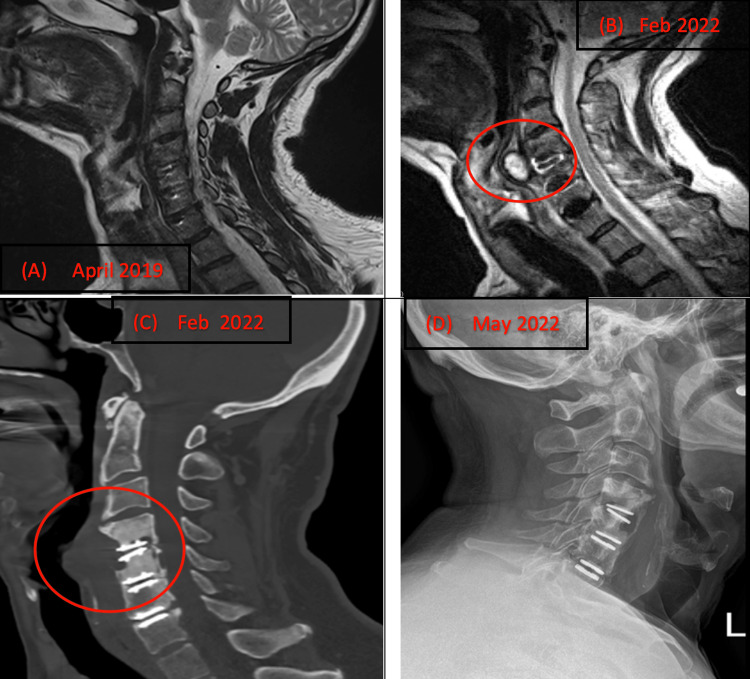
Images showing progressive changes seen within three years. (A) MRI of C4-C5 level shows sclerosis; (B) Cyst (red circle) is seen centrally anterior to the C4-C5 measuring 2.1 cm x 2.7 cm x 1.7 cm, right paracentral to the C4-C5 measuring 2.1 cm x 1.2 cm x 1.2 cm and left paracentral to the C3-C4 measuring 2.3 cm x 1.2 cm x 1.1 cm (not shown in the figure). (C) Mass effect on hypopharynx that is anteriorly displaced (red circle). Prevertebral soft tissue abnormality measuring 5.3 cm x 2.1 cm x 5.2 cm at the C4-C6 level.* *(D) X-ray: Osteolysis at the C4-C5 level with progressive loosening of the disc replacement hardware and progressive loss of bone around the devices at C4-C5 and to a lesser degree at C5-C6.
